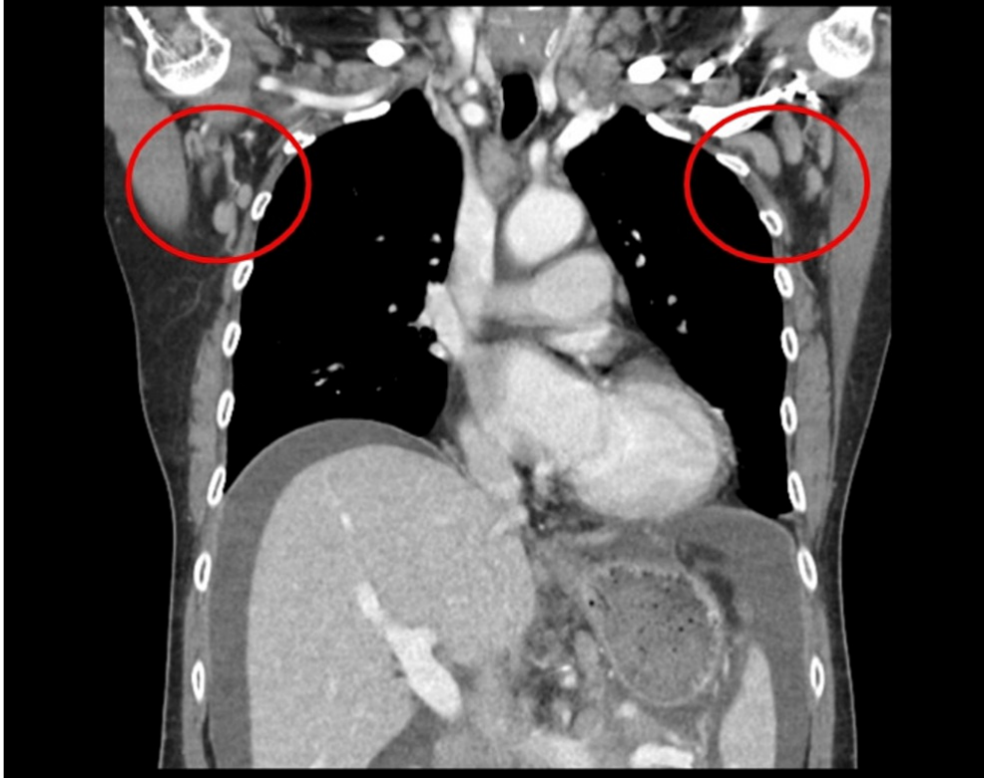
CT Chest with Contrast 2/9 showing bilateral axillary adenopathy (Red circles).
